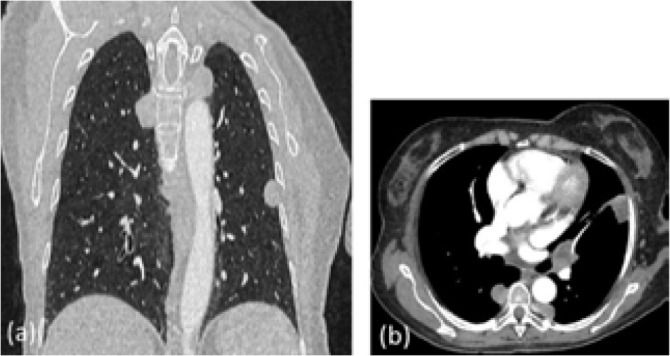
CT chest with contrast. (A) Coronal lung window (B) Axial soft tissue window. CT thorax with contrast demonstrating multiple rounded soft tissue lesions distributed throughout both lungs in keeping with lung metastases (white arrows).
